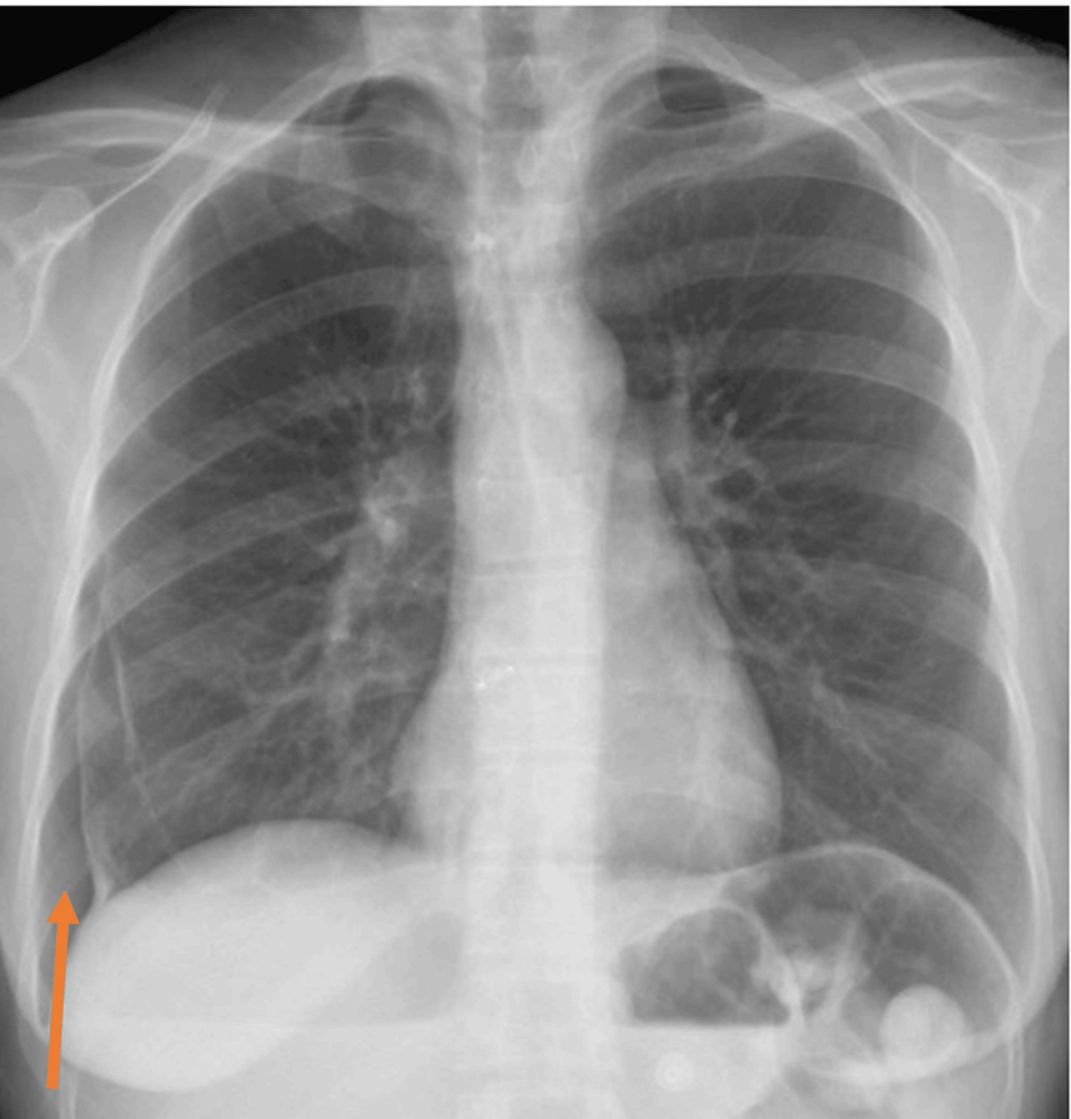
Chest radiograph Chest radiograph demonstrating a right-sided pneumothorax with no focal lung lesions or infiltrates. The absence of underlying lung pathology supports a spontaneous etiology
